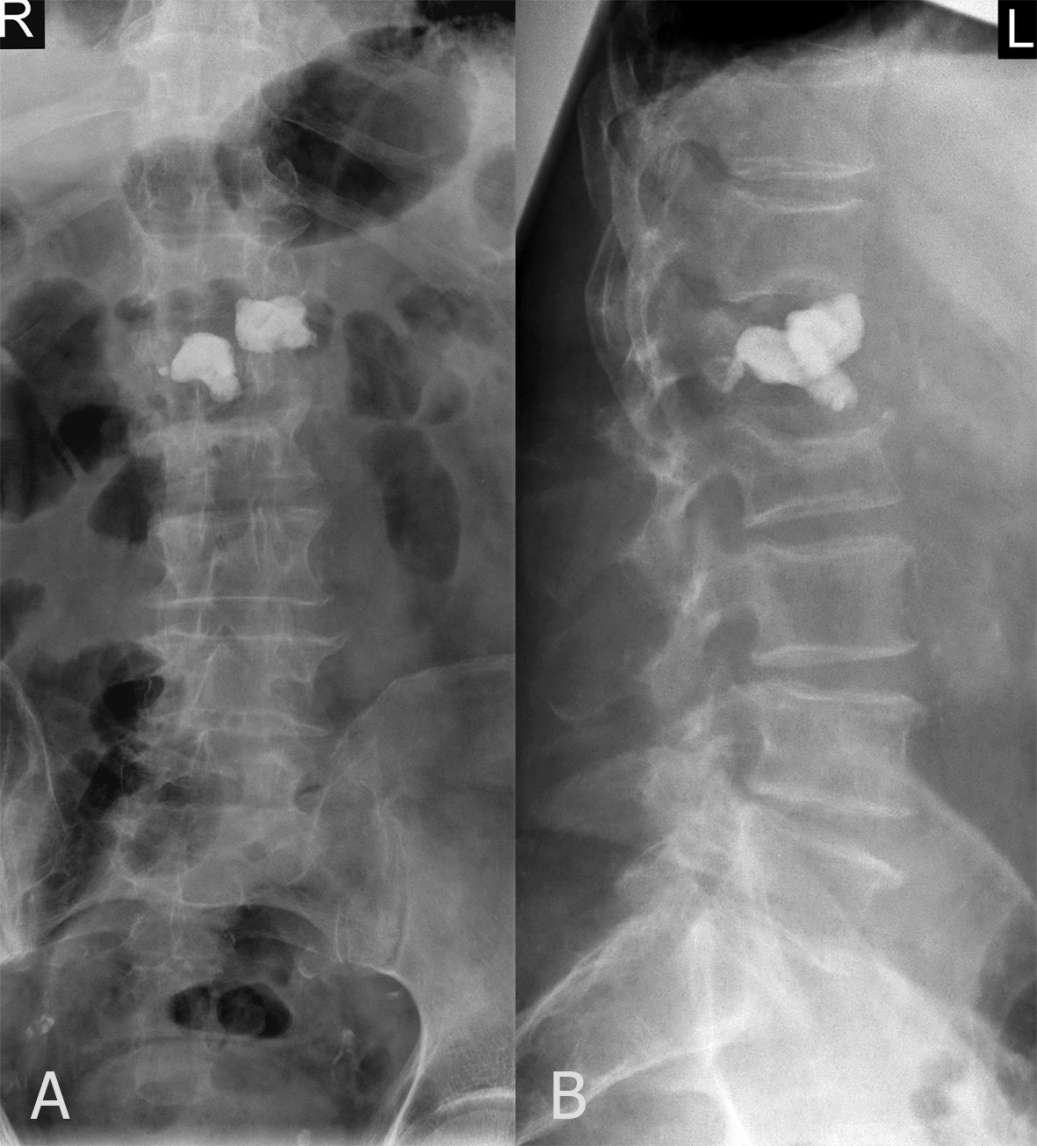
Anterior posterior (A) and lateral (B) thoracolumbar radiographs (T11 - S1) six weeks after initial kyphoplasty. The L1 vertebral body is partially resorbed. The osseous structure of the L1 vertebral body cannot be delineated. The position of the left cement block has shifted anteriorly and rostrally.
